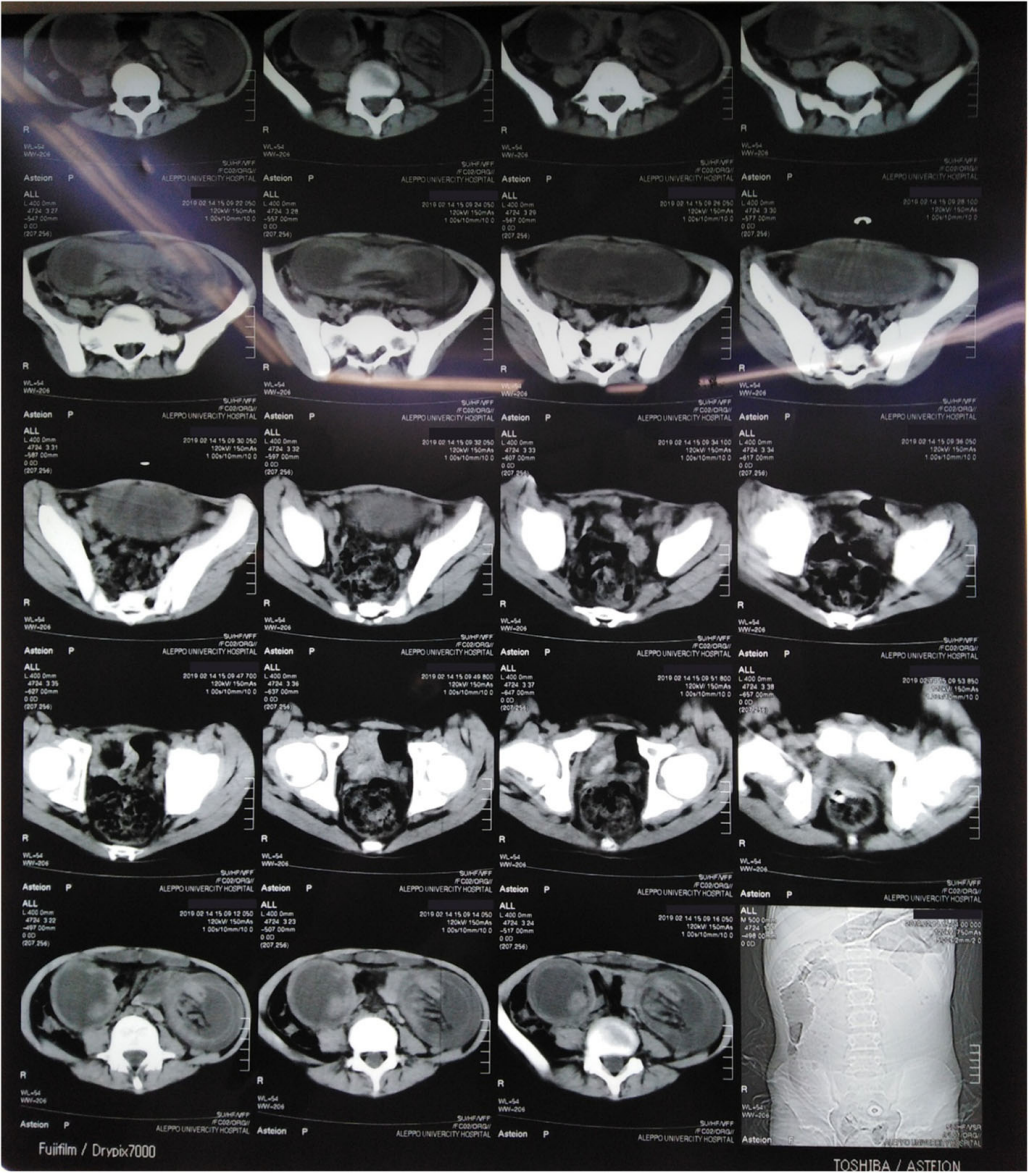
CT scan shows two bilateral abdominal lesions that may indicate dilated, invaginated intestinal loop
